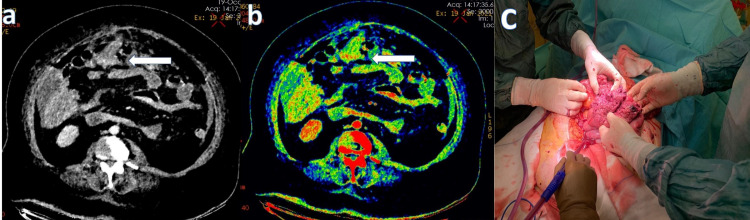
A 65-year-old female patient, who was operated on due to a malignant neoplasm of the corpus uteri, presented with abdominal pain and distension. In the IV contrast-enhanced dual-energy abdominopelvic CT examination, focal integrity loss in the small intestine in the midline of the abdomen and free air images were observed. Transmural loss of wall enhancement on (a) 45 keV virtual monoenergetic images (white arrow) and (b) iodine overlay map images (white arrow). (c) Perforation localization in the jejunal segment covered with necrotic tissue.
